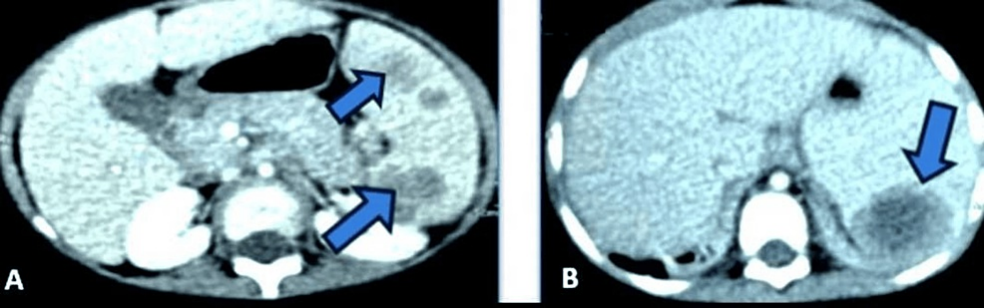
Thoraco-abdominal CT scan (A and B) showing the liver increased in size, with regular contours and homogeneous density, an enlarged spleen, heterogeneous, multiple lesion sites, well-limited, heterogeneous hypodense, necrotic, and heterogeneously enhancing after the injection of contrast medium.
